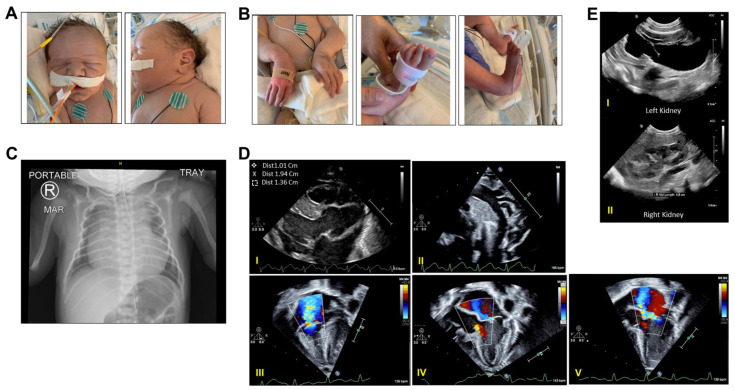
Clinical features of proband A suggesting neonatal Marfan syndrome. (A,B) Representative photographs of proband A face (A) showing and extremities (B). (C) Representative chest X-ray on day of life 2 (DOL2) showing cardiomegaly and abnormal rib cage. (D) Representative echocardiography images on DOL 2. (I) Parasternal long axis view showing dilated aortic root (Z-score: +5.7); (II) normal left aortic arch with normal pattern branching; (III) Color Doppler (CD) interrogation across the tricuspid valve (TV) showing tricuspid regurgitation; (IV) CD interrogation across the aortic valve showing mild aortic insufficiency; and (V) CD interrogation across the mitral valve (MV) showing mild eccentric MV regurgitation, a feature commonly seen in dysplastic valves. Also, refer to supplemental Video S1. (E) Representative kidney ultrasound images on DOL 2. (I) Left kidney hydronephrosis SFU grade 3; (II) right kidney.
